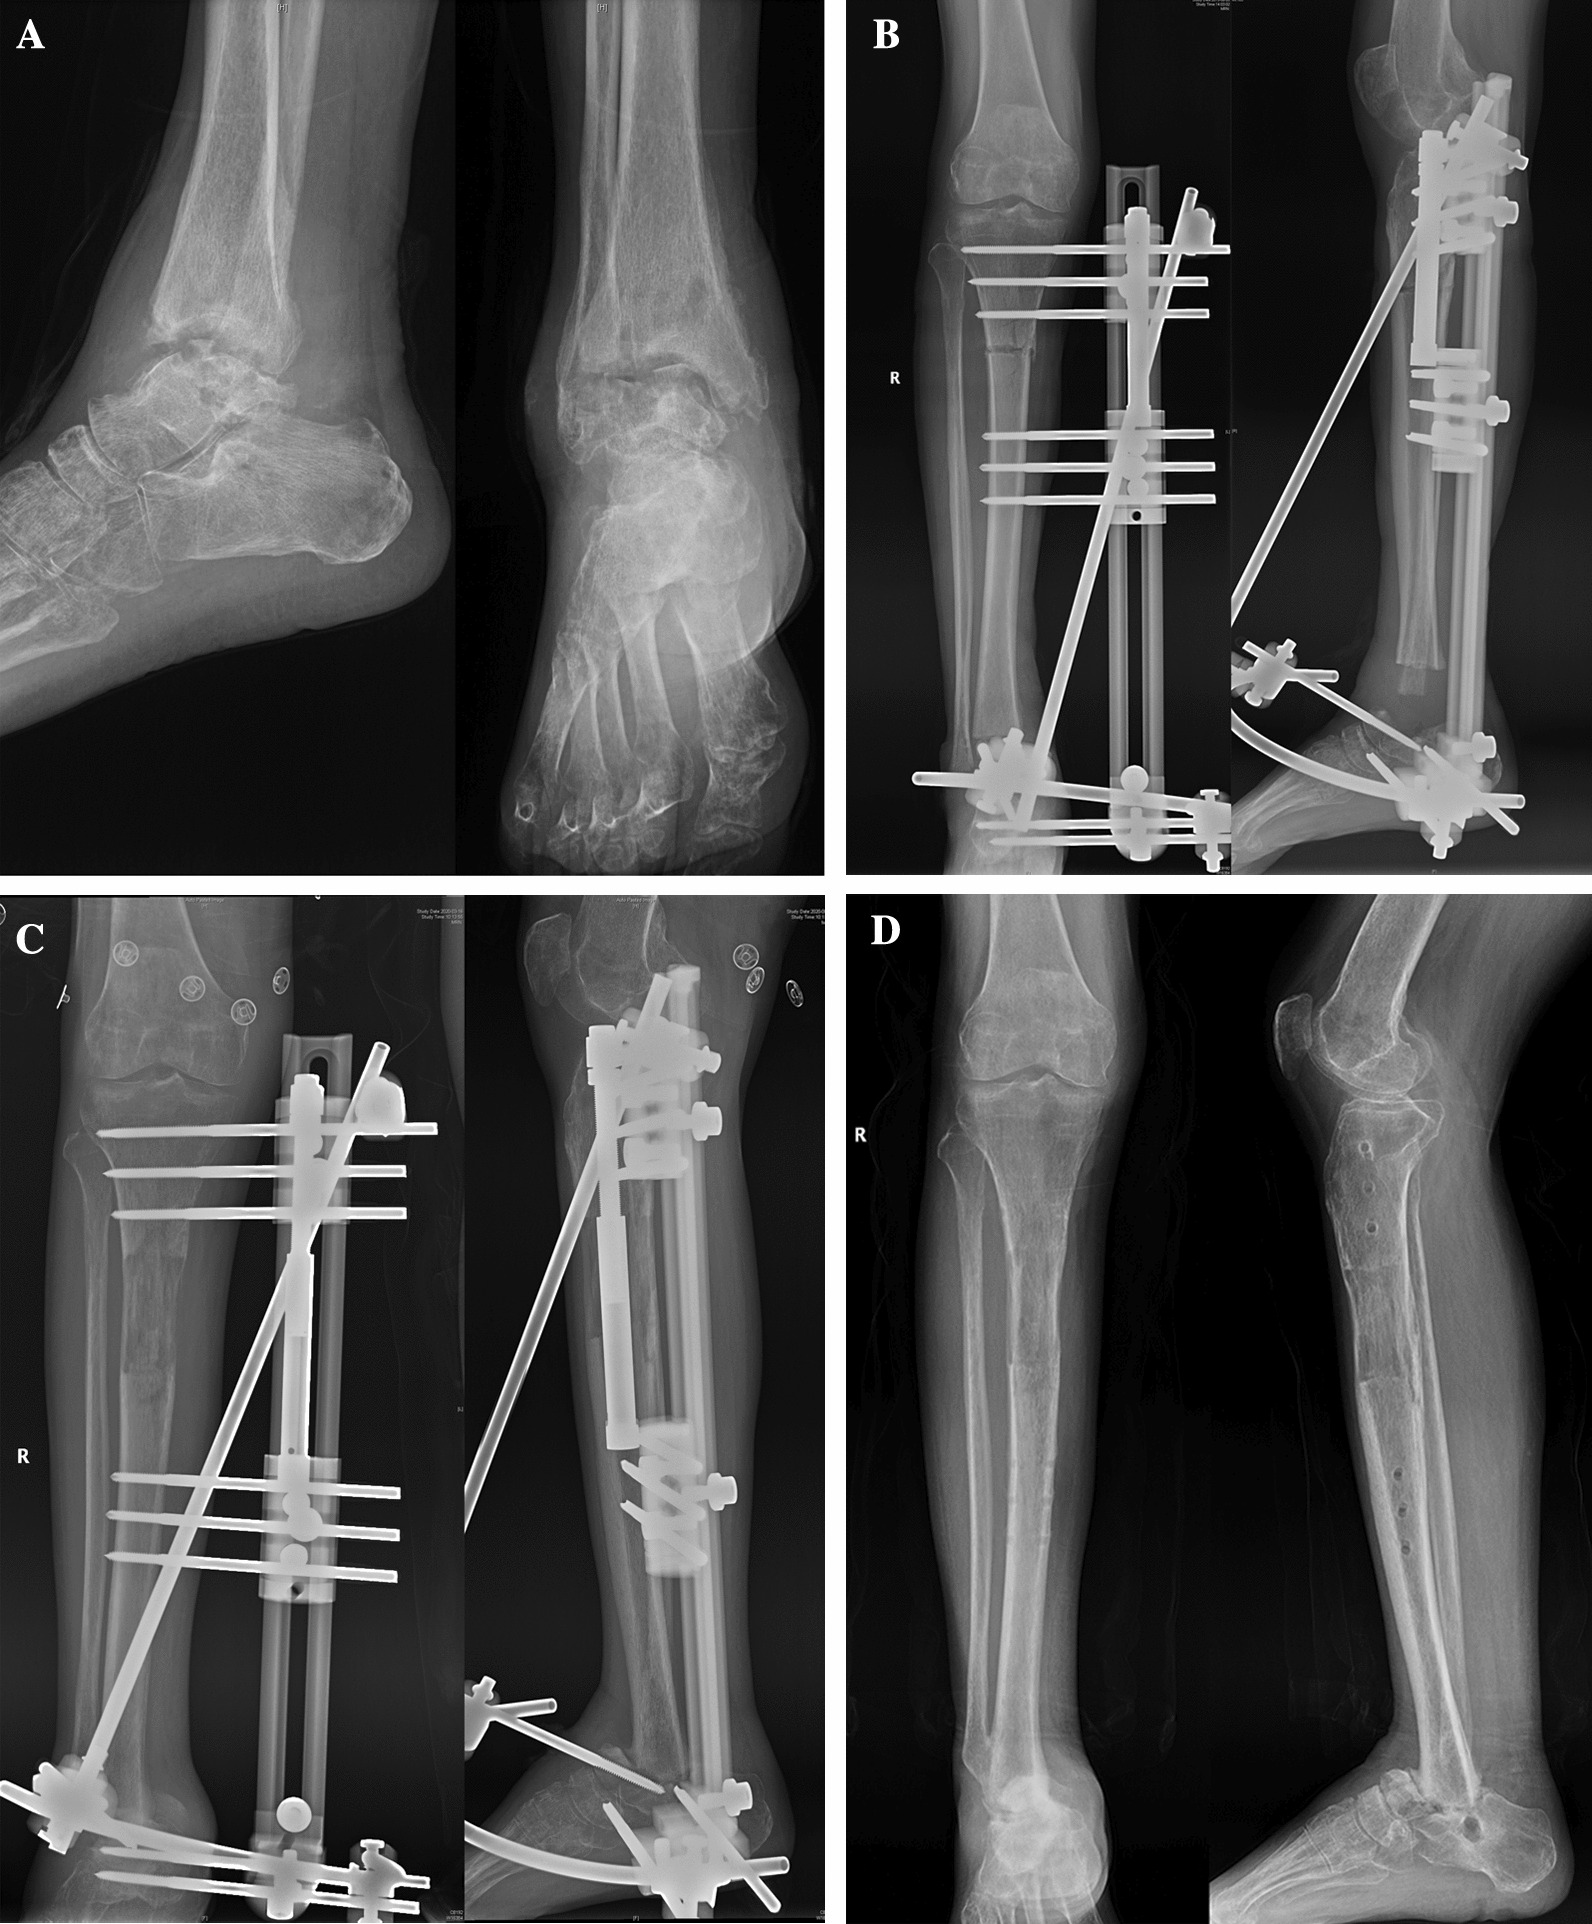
A. X-ray showed the infection of the talus and the infection-related bone destruction had spread the whole ankle joint. B. Postoperative X-ray showed the complete removal of the infected lesions using unilateral external fixation and osteotomy. C. The noticeable mineralization of the new bone within the bone transport segment could be achieved. D. Successful tibio-talar fusion and excellent mineralization of the new bone could be achieved. Additionally, the external fixator was removed
